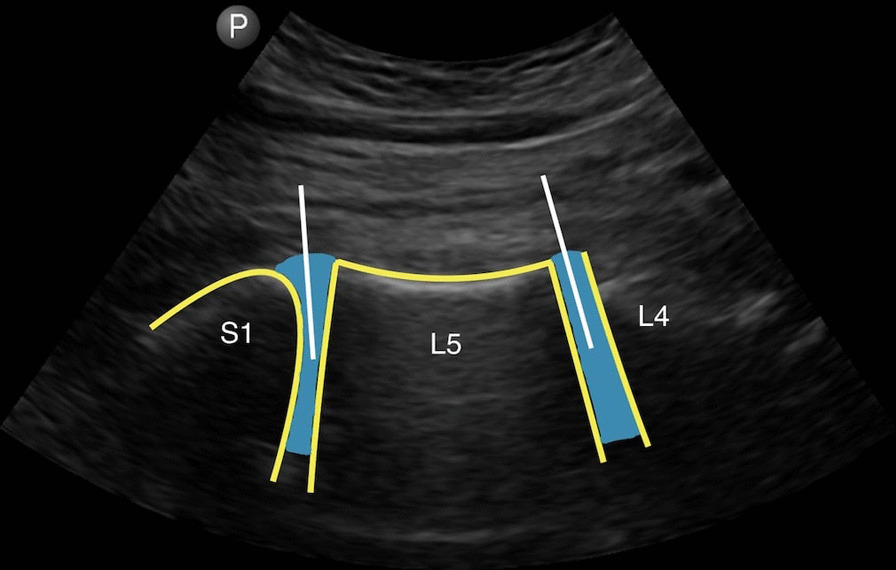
Image of L4–S1 with ultrasonic long-axis scanning
